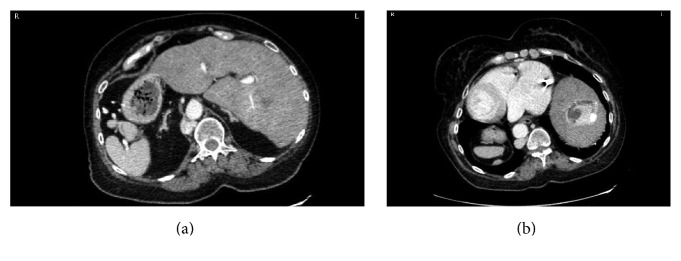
((a) and (b)) Transverse CT images of angiomyolipoma in a patient with situs inversus totalis.
